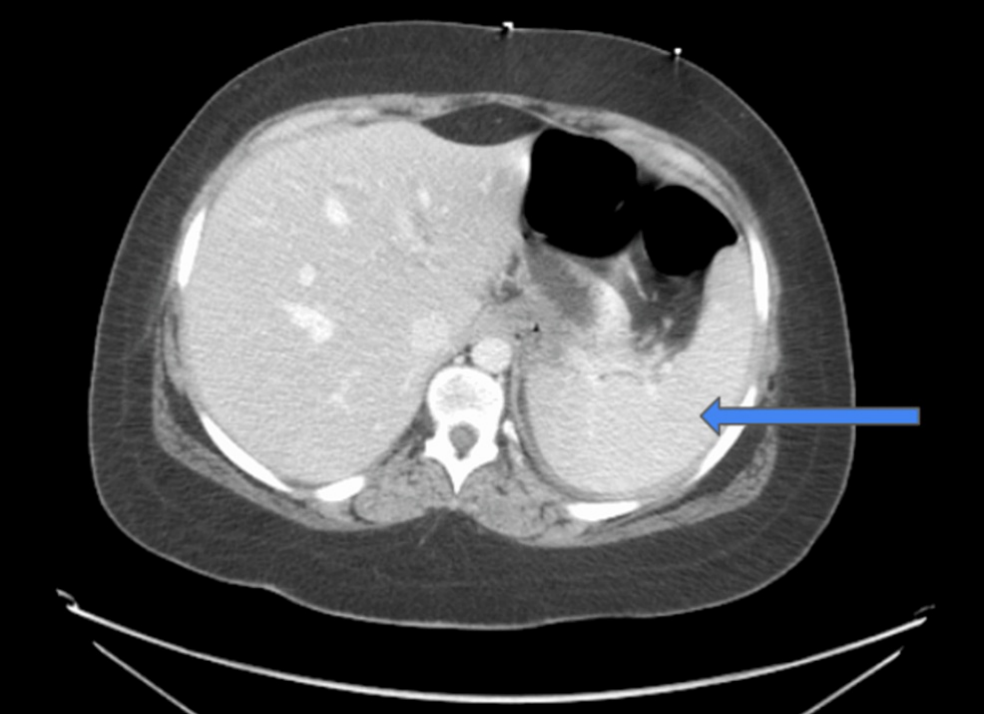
CT of the abdomen/pelvis with IV contrast at presentation showed mild splenomegaly (blue arrow)
